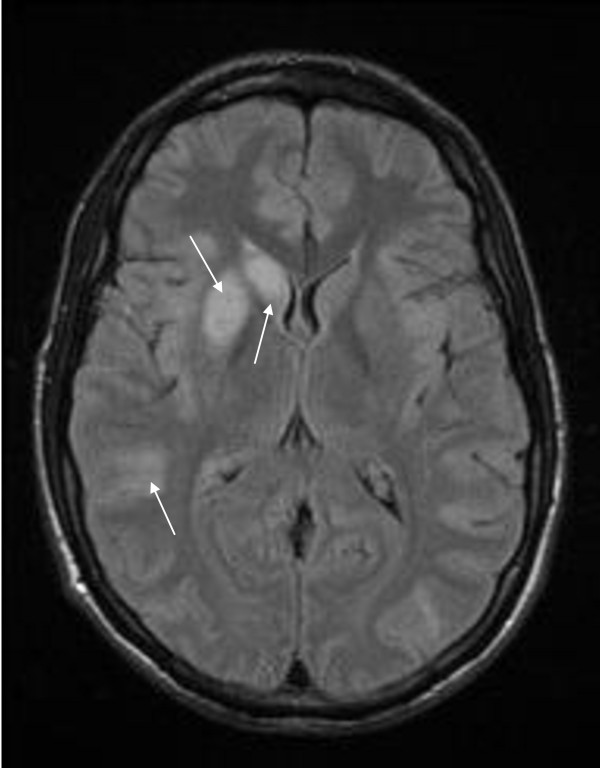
T2-weighted magnetic resonance imaging scan showing bilateral superficial and deep to the right ischemic infarcts which concern different vascular territories in a young adult four days after he smoked cannabis.
